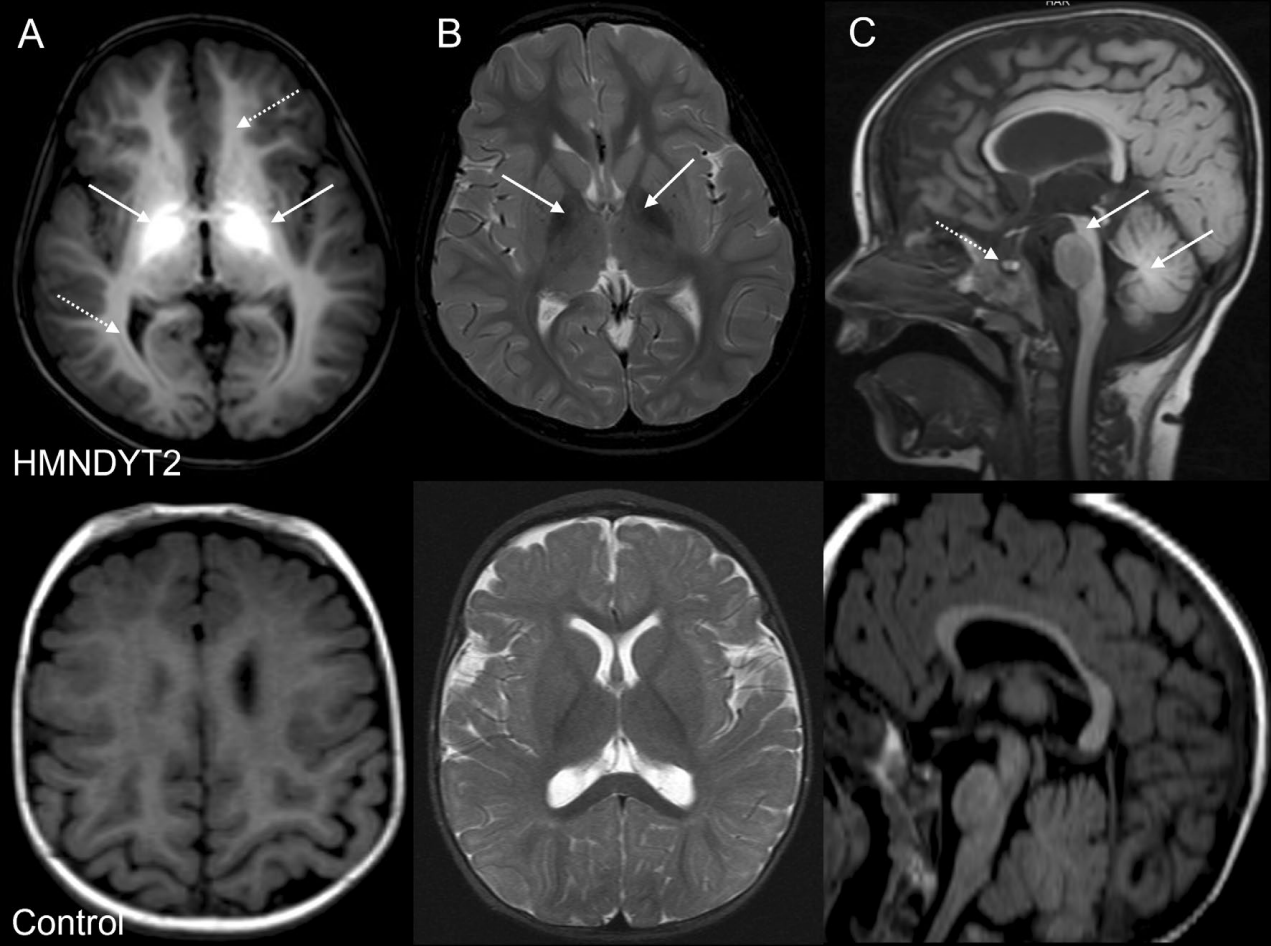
Characteristic MRI brain appearances of manganese deposition. Representative images from a 10 month old patient with HMNDYT2 (top row) compared to a healthy control subject (bottom row). (A) T1‐weighted imaging, axial view: T1‐weighted hyperintensity marked by a white arrow pointing to the globus pallidus, dashed arrow pointing to the white matter. (B) T2*‐weighted imaging, axial view: Corresponding hypointensity marked by a white arrow pointing to the globus pallidus. C T1‐weighted imaging, sagittal view: White arrows pointing to dorsal pons and cerebellar peduncles, dashed arrow pointing to T1 shortening in the anterior pituitary gland.
